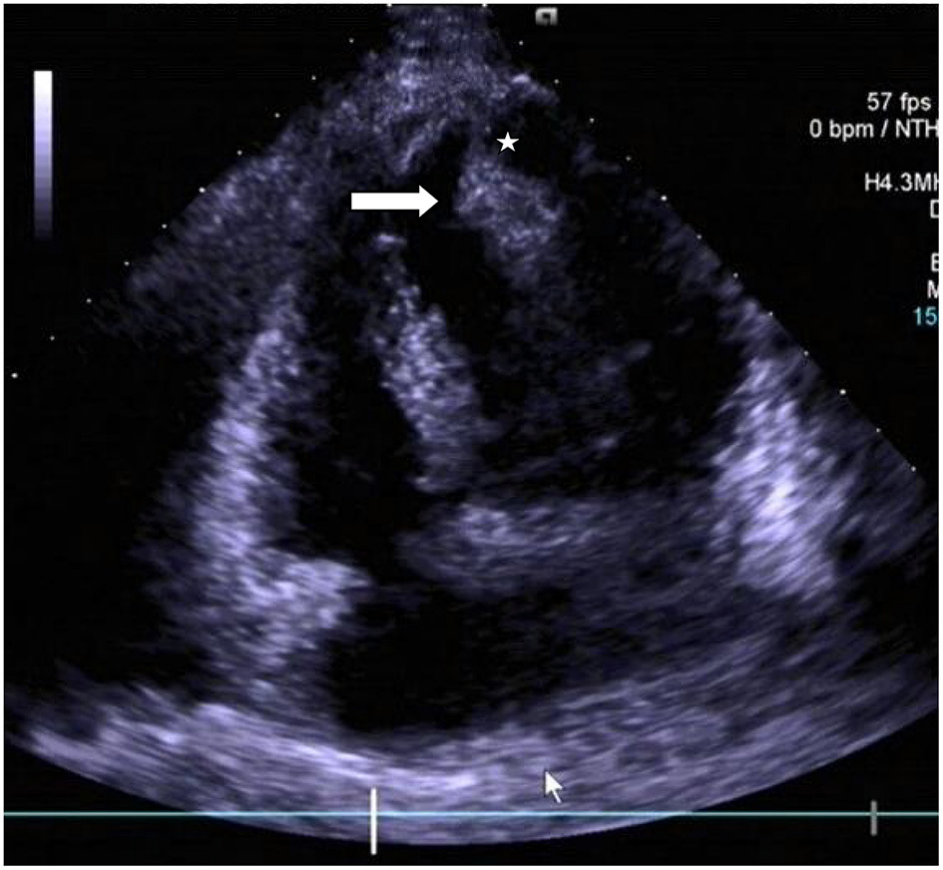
Transthoracic echocardiogram revealing the presence of a pedunculated lobular mass [peduncle (asterisk); mass (arrow head)] within the left ventricle.
